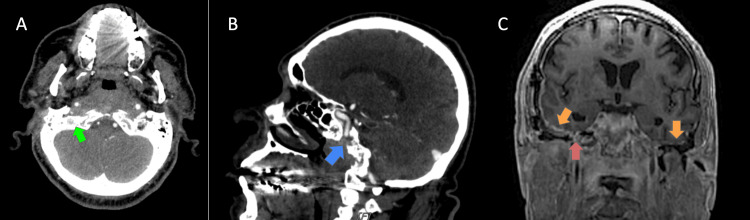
CT head of the patient and MRI of the IACs A: Sclerosis of skull base consistent with sequelae of osteomyelitis (green arrow) B: Right temporal postsurgical changes (blue arrow) with no acute findings C: MRI of the IAC reveals persistent skull base osteomyelitis (red arrow) extending from the right to the left temporal bone, dural thickening and enhancement (orange arrow) along both petrous pyramids and walls of both IACs IAC: Internal auditory canal
